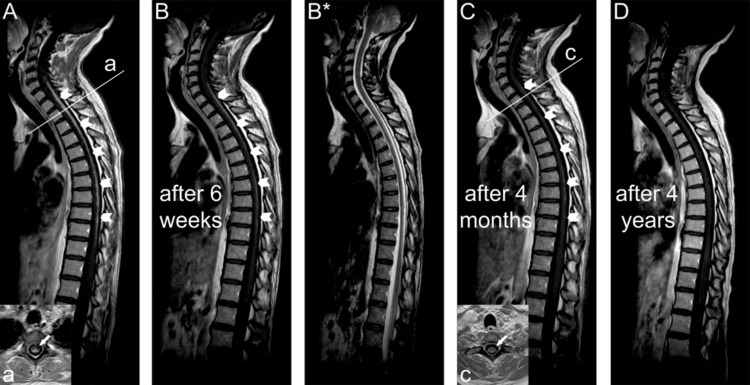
Longitudinal spinal cord lesion with trident sign in neurosarcoidosis before and after treatment Spinal MRI showed dorsal subpial and intramedullary gadolinium enhancement in T1-weighted sequences across 12 vertebral segments from C6 to T10 [A, marked by arrowheads; "a": cross-sectional image at the T2 level with the trident sign (marked by an arrow)]. The subsequent MRI scans showed complete reduction of the spinal lesion within the next four years [B-D, marked by arrowheads in B and C; B* shows the corresponding T2-weighted sequence from the same time point as in B; "c": cross-sectional image at the T1 level, with the trident sign no longer visible (arrow)].
